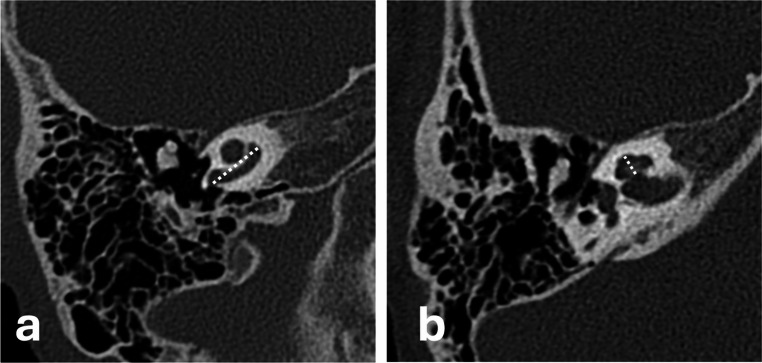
Measurement parameters of cochlea on axial CT images for cochlear hypoplasia. (a) Basal turn length is measured along the basal turn of cochlea at the level of round window. (b) Mid-modiolar height is measured as the distance from cochlear apex to the base of modiolus. Cochlear hypoplasia is considered when the basal turn length and mid-modiolar height measure less than 7.5 mm and 3.4 mm respectively
